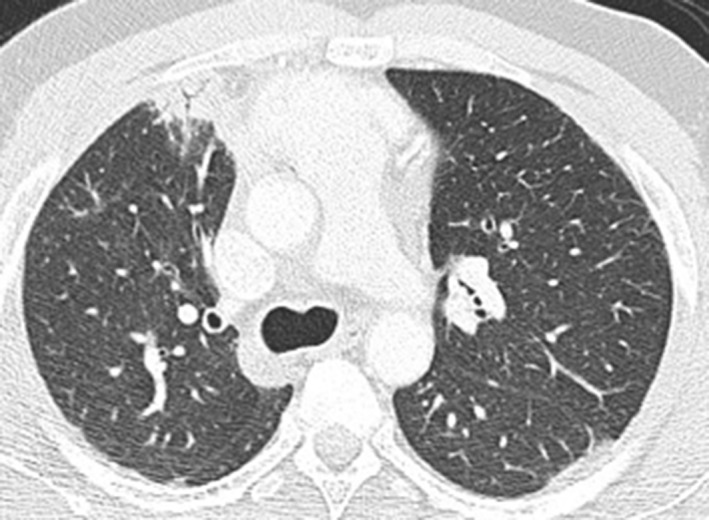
CT imaging demonstrating nonspecific patchy sub‐pleural consolidation anteriorly in the right upper lobe and in the apical segment of the left lower lobe.
